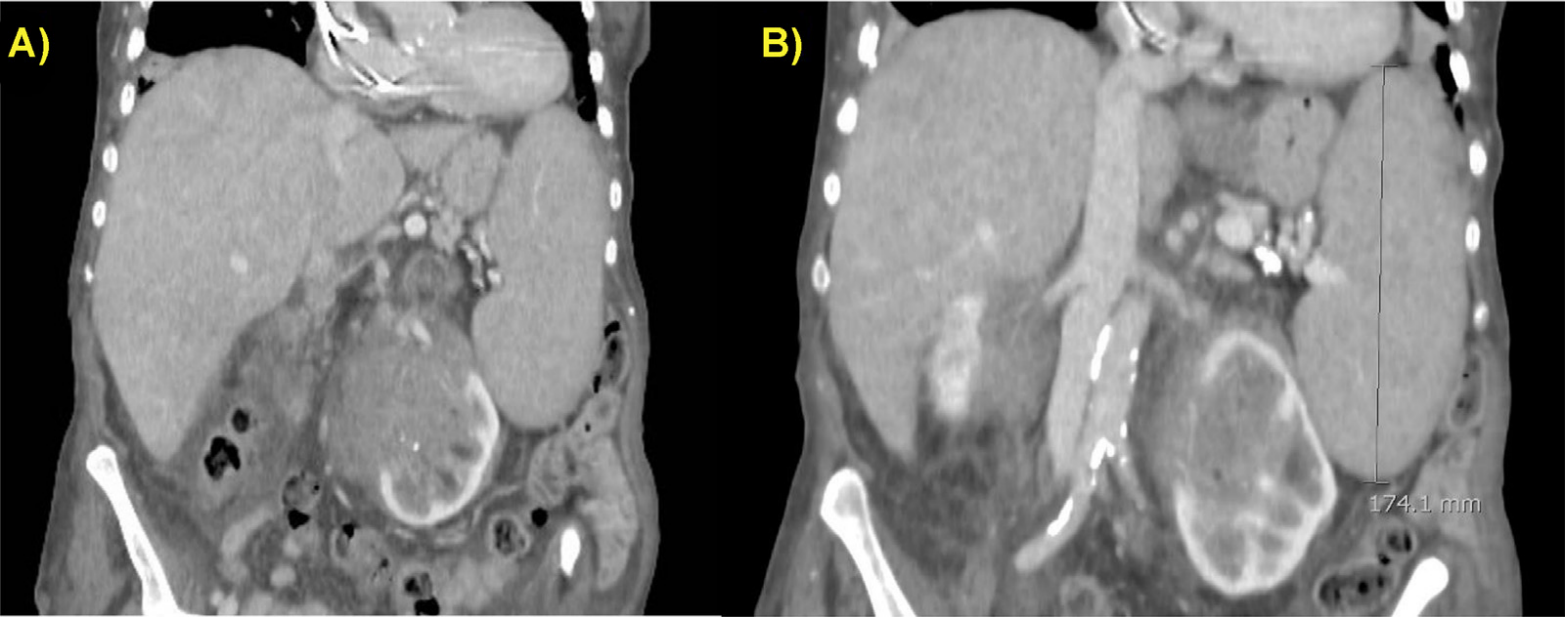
(A and B) Contrast-enhanced CT scan on coronal planes showed the left kidney hemorrhage (A) with concomitant splenomegaly (maximum diameter of 17 cm) and liver enlargement.
